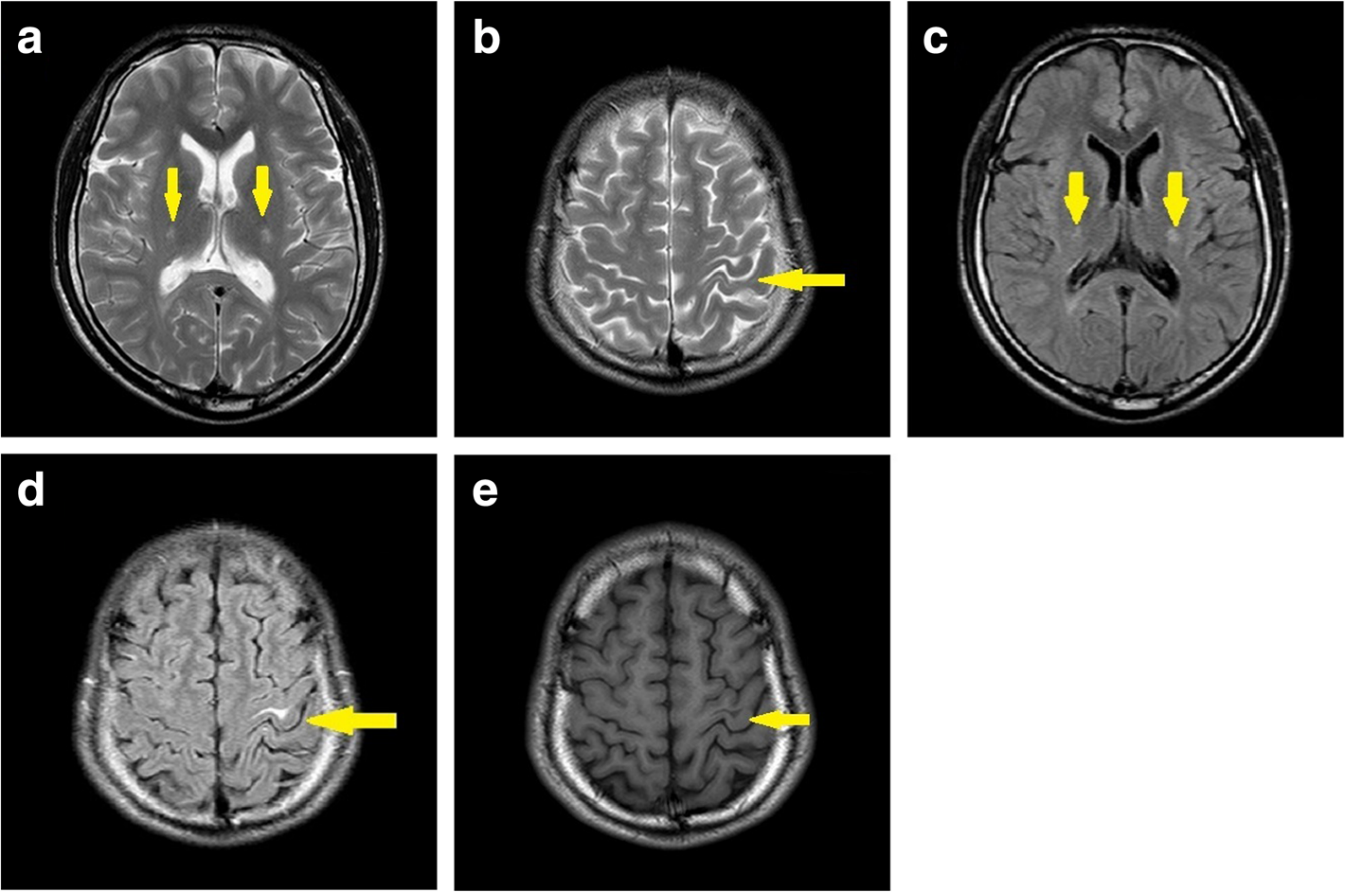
Cerebral MR scans of the patient 1. The axial T2w and FLAIR image showed bilateral corticospinal tracts (a and c) and the left central gyrus cortex (b and d) signal hyperintensities. Cerebral T1w axial images (e) revealed a decreased signal in the left central gyrus cortex
